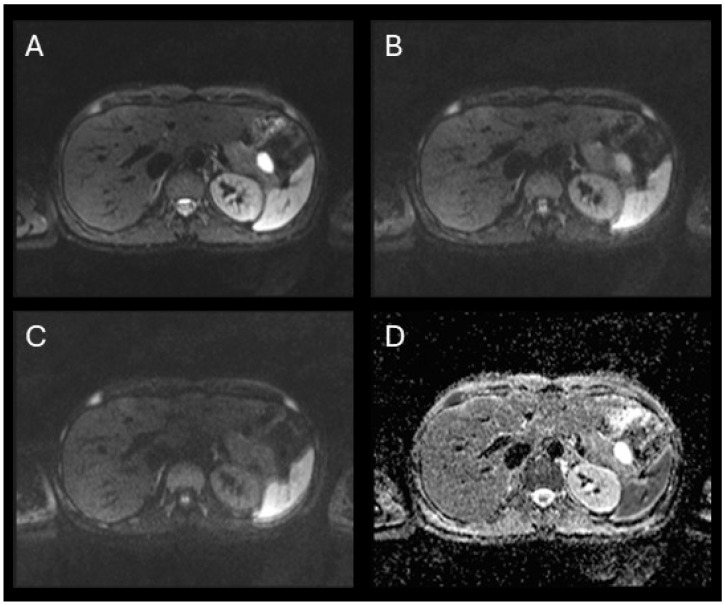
Axial diffusion-weighted MRI of the abdomen evaluating the cystic lesion in the pancreatic tail. (A–C) DWI images at b-values of 50 (A), 400 (B), and 800 s/mm^2^ (C) show progressive signal loss with increasing b-values. The lesion demonstrates high signal on b50 due to T2 shine-through, with marked signal attenuation by b800, indicating free diffusion. (D) The corresponding ADC map shows high signal intensity within the lesion, confirming the absence of restricted diffusion. These findings are consistent with a benign cystic lesion, such as cystic pancreatic hamartoma.
